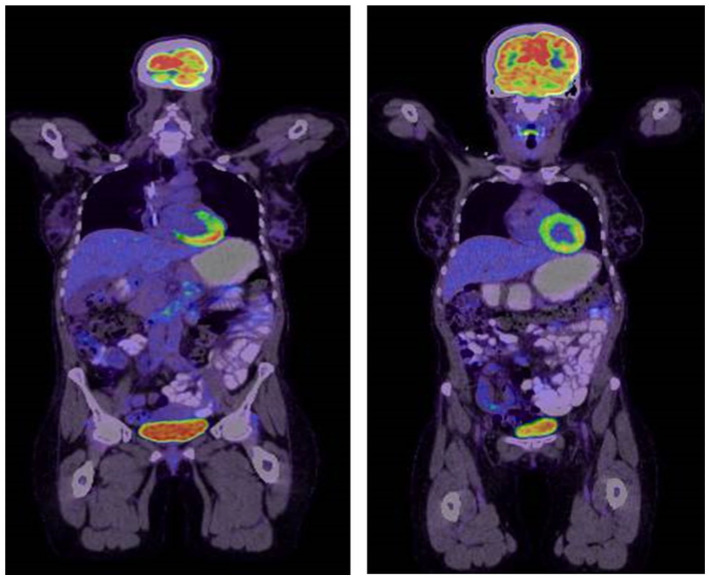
PET–CT scan showed complete response with no suspicious uptake, suggestive of complete response. PET–CT: positron emission tomography–computed tomography.
